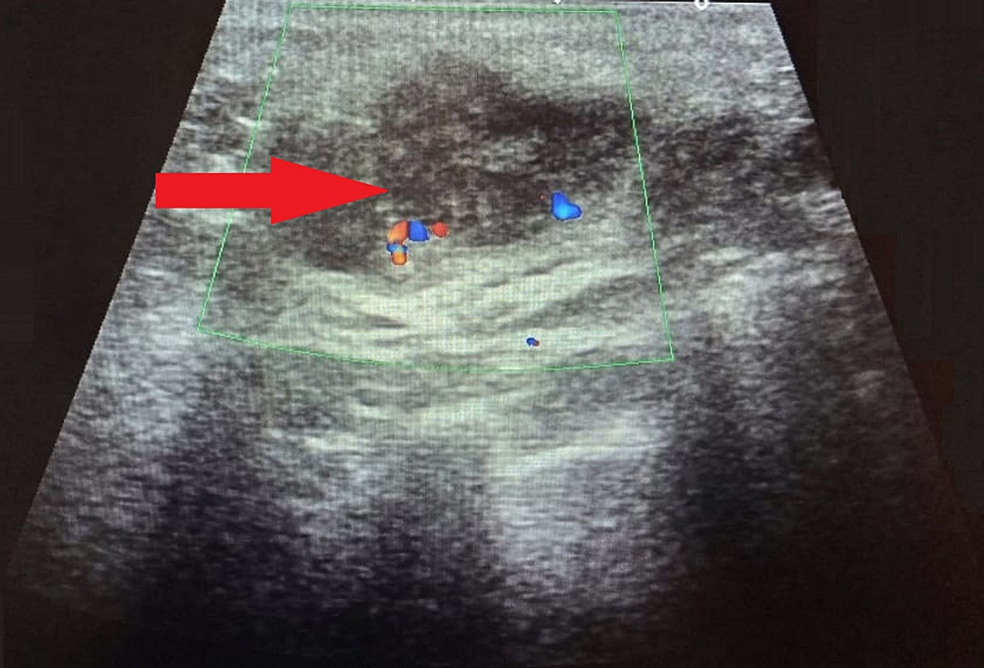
Ultrasonography of the breast showing an ill-demarcated hypoechoic irregular lesion with no fluid components. The area of pathology is marked by a solid red arrow.
